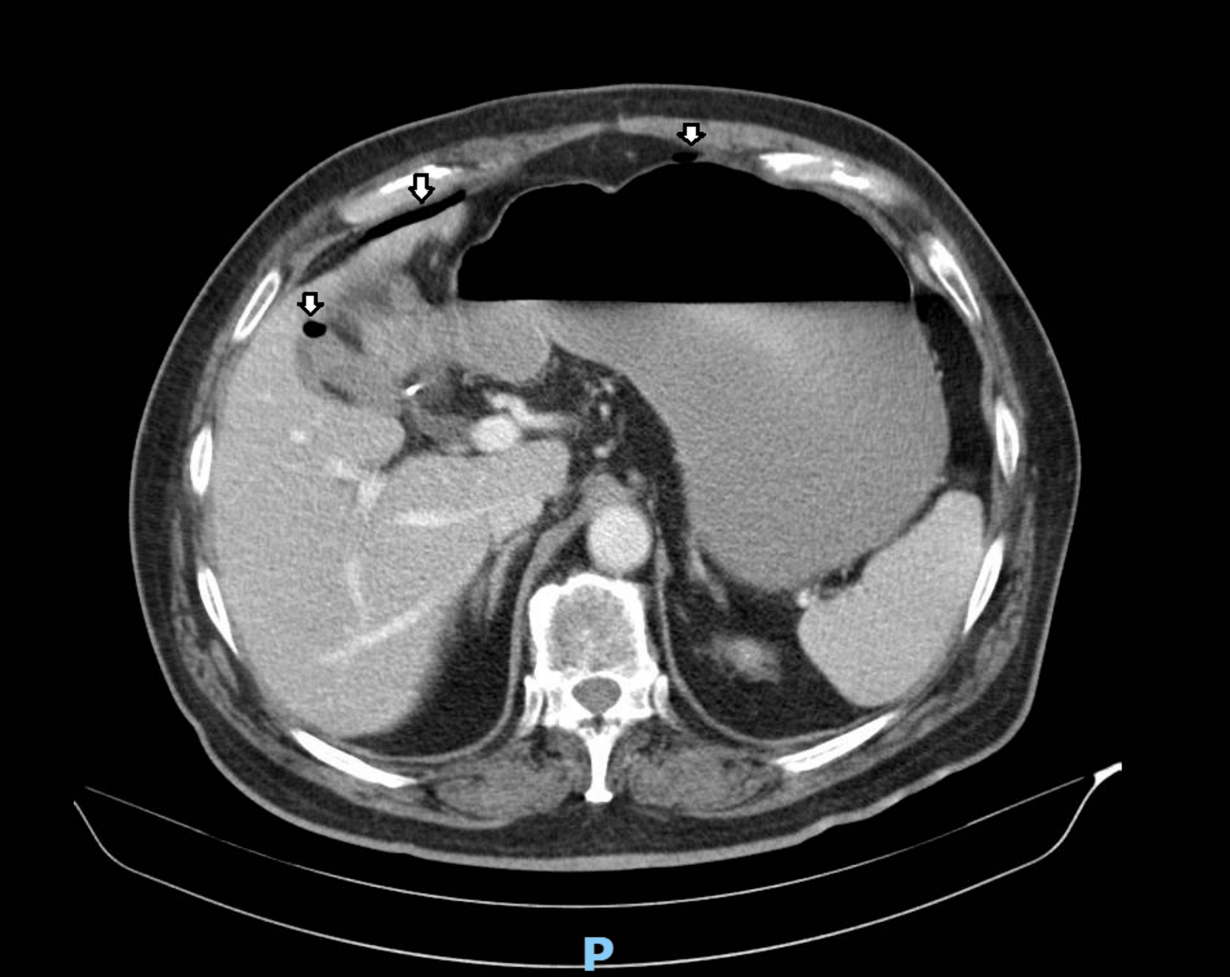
CT scan revealing pneumoperitoneum
